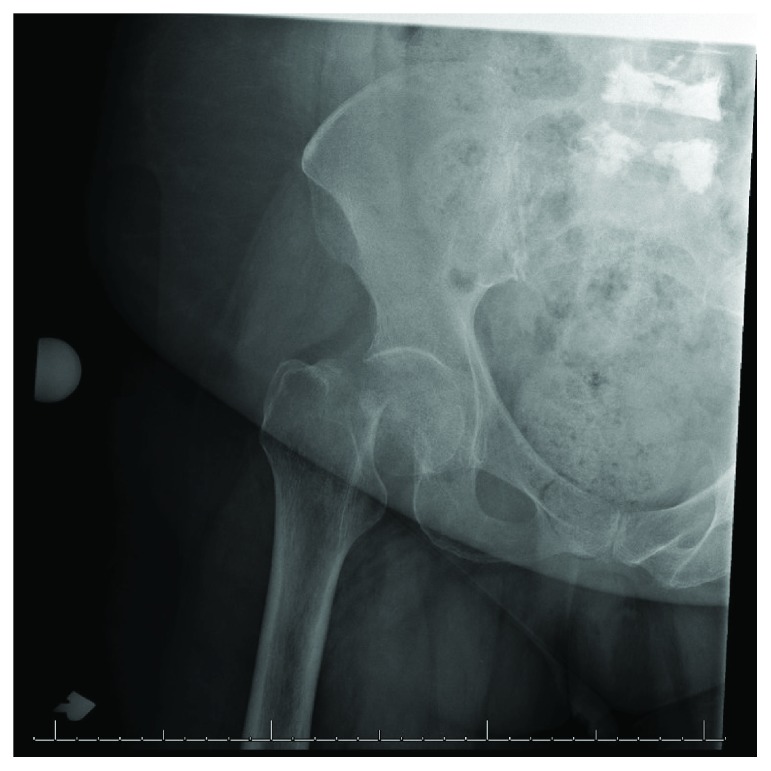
AP radiograph of the right hip demonstrating a displaced femoral neck fracture.
